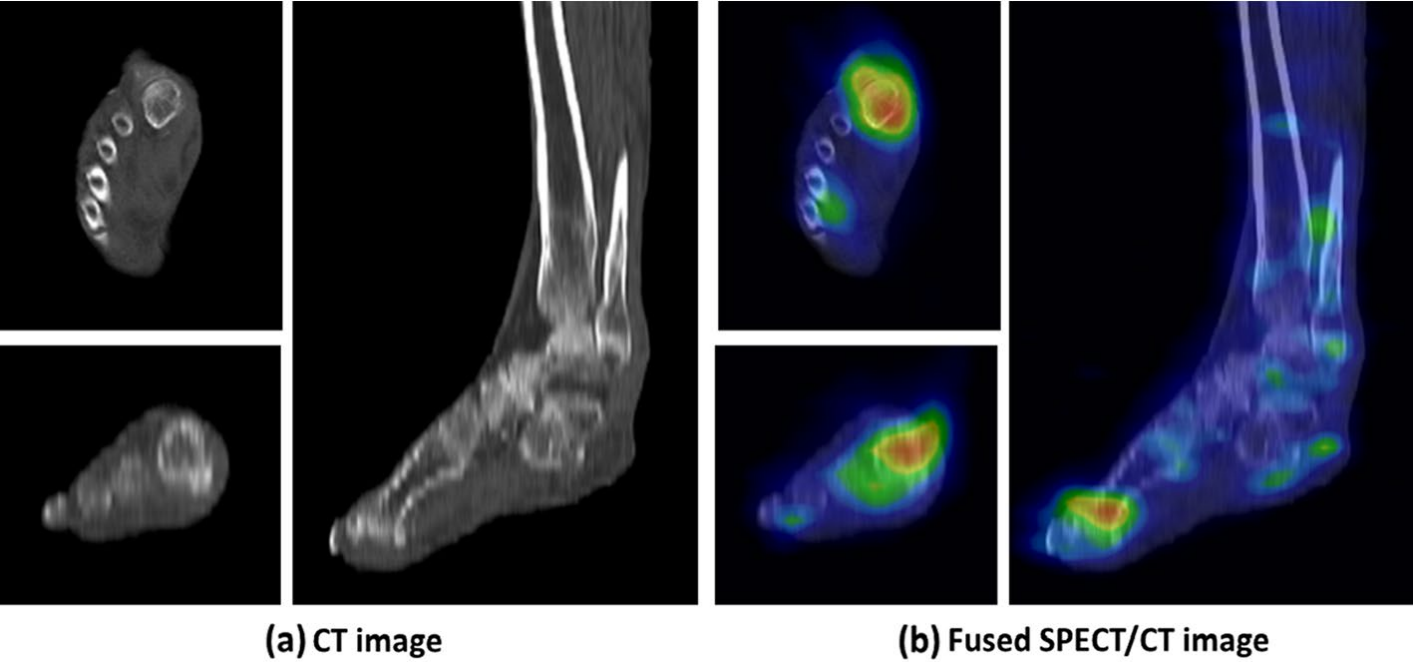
These images represent a 68-year-old man with diabetic gangrene, hypertension, and atherosclerosis obliterans as comorbidities. a shows CT images indicating increased subcutaneous density and accumulation indicative of cellulitis near the right 1st proximal phalanx, and metatarsal bone with irregular bone destruction, and increased attenuation lesions in the subcutaneous tissue. b shows fused SPECT/CT images indicating distinct accumulation in the subcutaneous tissue, right 1st proximal phalanx and metatarsal bone with high LBR (12.00) and TLU (133.76) and low SUVmax (3.45). The primary physicians diagnosed this patient with CE. The final diagnosis of LLOM was confirmed by the pathologist. The findings indicated sequestrum. Thirty-nine days after the scanning, the patient experienced a fatal event due to sepsis
